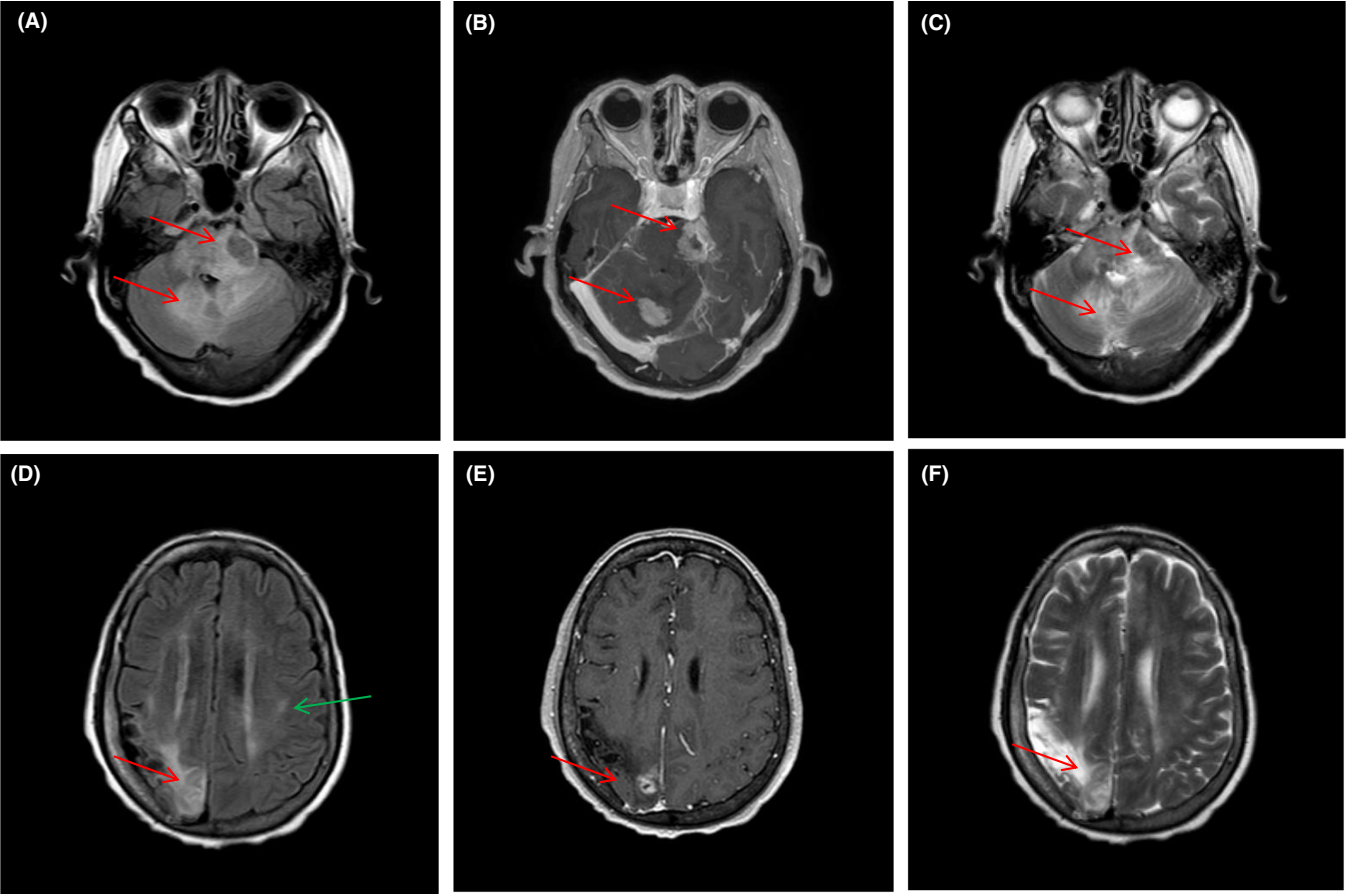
Pre‐treatment magnetic resonance imaging with ill‐defined lesion in brainstem, cerebellum (A, B T1‑weighted axial; C, T2‑weighted axial). T1‑weighted axial (D, E) and T2‑weighted axial (F) images showing enhancing lesion in the cerebral. (Red arrow means NCS involvement, green arrow means ischemia strokes).
